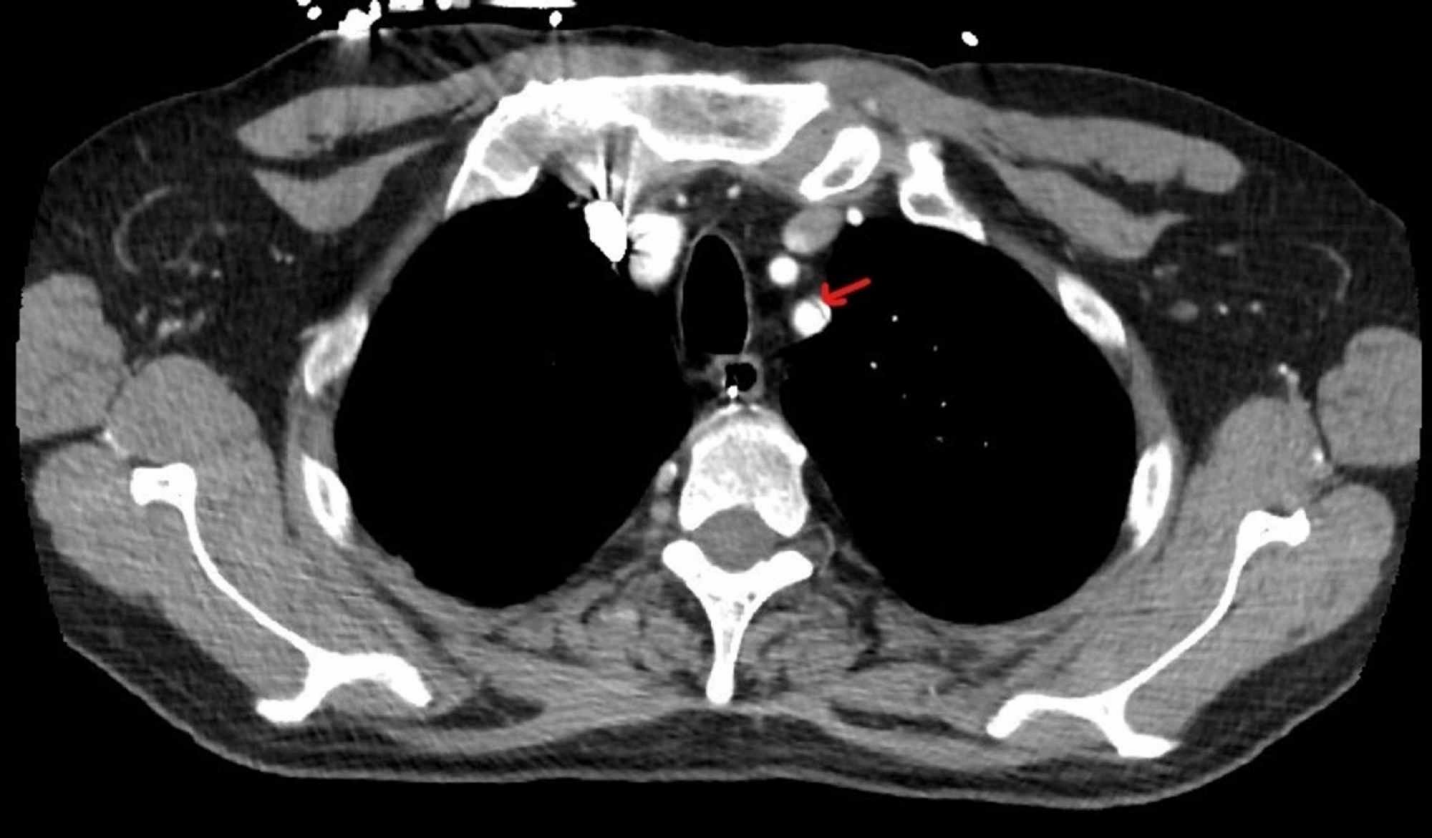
Left subclavian artery dissection (SAD) without involvement of the aorta or branch vessels on computed tomography angiogram (CTA) scan.
